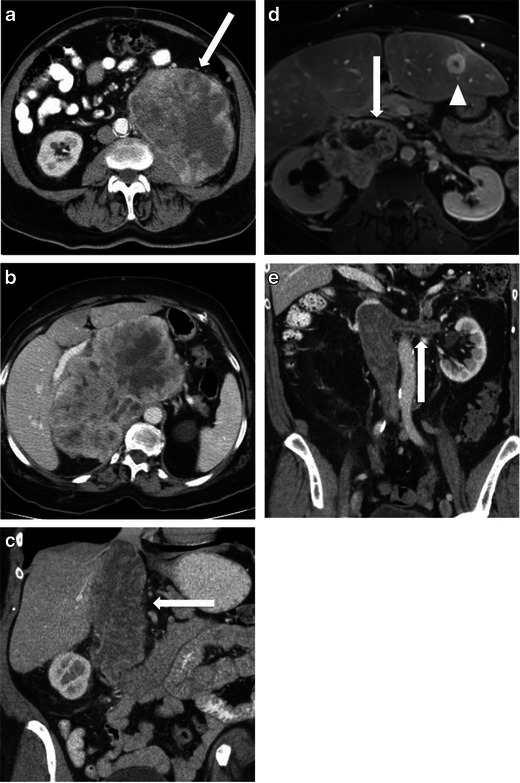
Imaging of leiomyosarcoma. a An 85-year-old man who presented with abdominal pain. CT shows a large heterogeneous left sided retroperitoneal mass (*arrow*) with central low attenuation in keeping with necrosis. b A 64-year-old woman presenting with early satiety. CT shows a large heterogeneous in the right abdomen, which is displacing the overlying liver. c, d A 60-year-old woman with IVC leiomyosarcoma. Coronal contrast-enhanced CT image (c) shows a large mass within the IVC (*arrow*). Axial T1-weighted image post gadolinium in the same patient (d) shows a heterogeneous enhancing mass centred on the IVC (*arrow*) and extending beyond the IVC into the paracaval location. There is moderate degree right-sided hydronephrosis secondary to the mass. Liver metastasis is noted in segment III of the liver (*arrowhead*). e A 77-year-old woman with IVC leiomyosarcoma extending to *left* renal vein. Coronal CT shows a heterogeneous enhancing mass filling the IVC and extending into the left renal vein (*arrow*)
